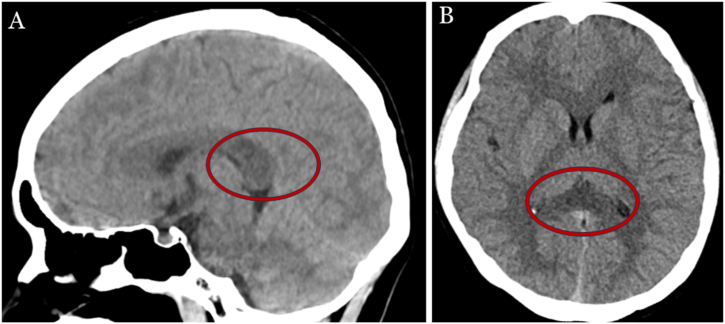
Head CT on admission showed abnormal hypodensity lesions in the SCC (A, B).
